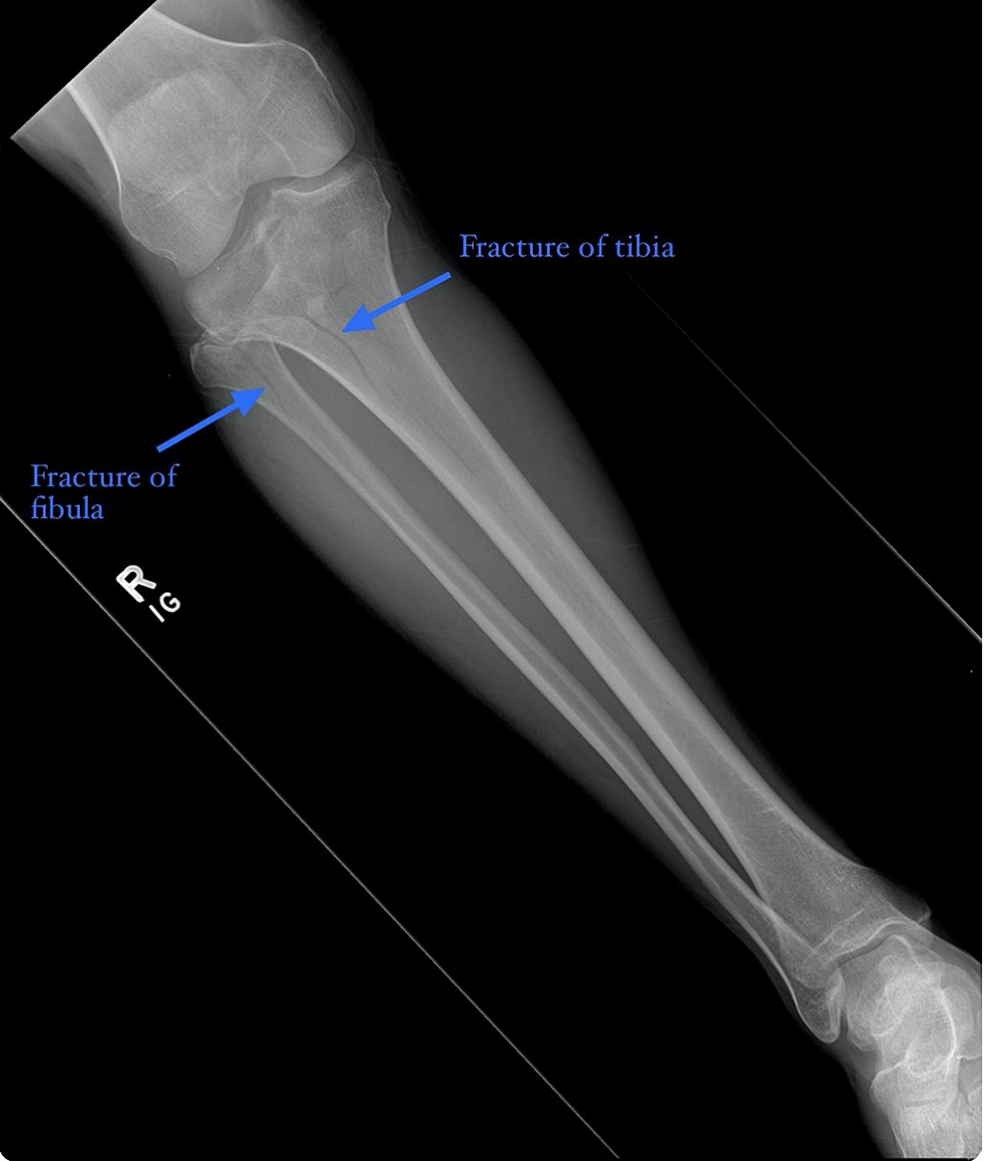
Plain radiograph of the right tibia fibula showed comminuted proximal tibial and fibular fractures (blue arrows) with evidence of intra-articular extension, right knee joint effusion, and lipohemarthrosis
